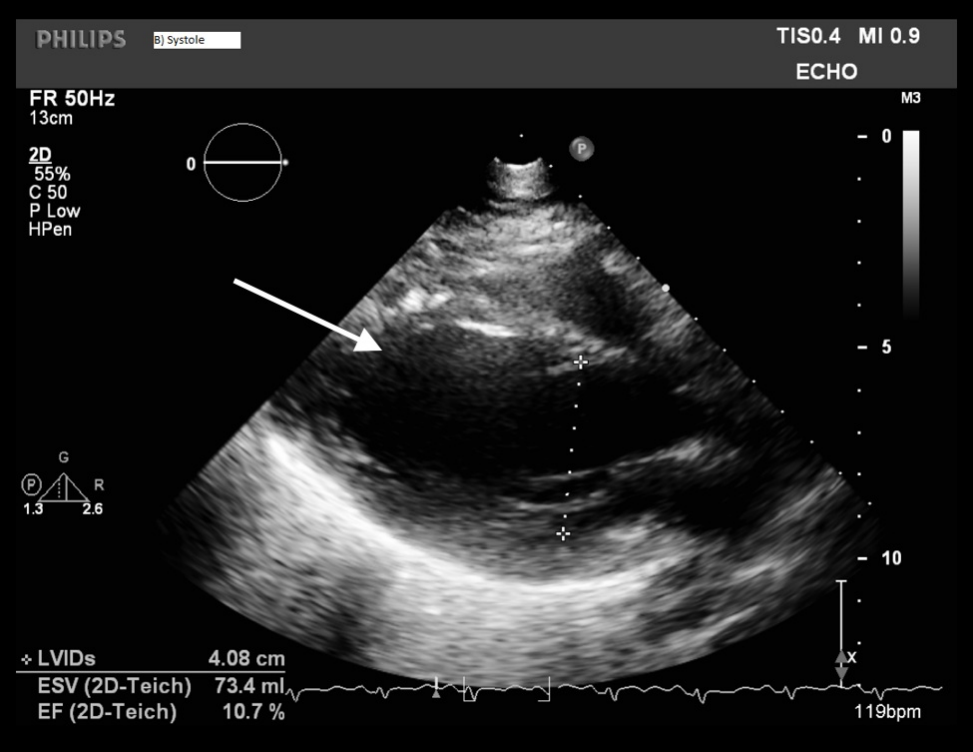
Initial TTE obtained at the time of admission showing an estimated ejection fraction of 10-15%. The arrow shows the dilated left ventricular chamber. TTE - Transthoracic echocardiogram
